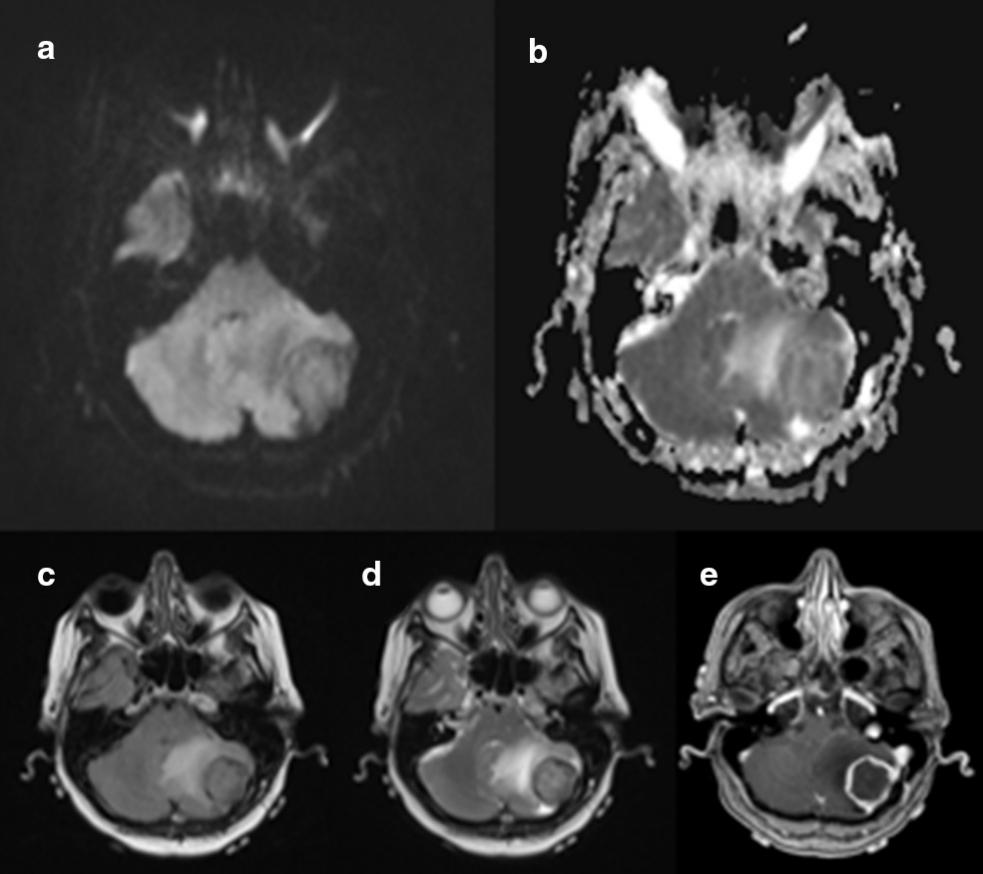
MRI scan of Case 1 demonstrating an extra-axial well-circumscribed mass within the left posterior fossa with a dural base against the left occipital bone. Diffusion-weighted image (DWI) (a) and associated apparent diffusion coefficientimage (b) demonstrate no restriction in diffusion. Fluid attenuation inversion recovery (c) and a *T*_2_ weighted image (d) shows associated increased signal consistent with peritumoral oedema and subsequent mass effect and effacement of the fourth ventricle. Contrast enhanced *T*_1_ image (e) shows a marginally enhancing lesion with dural tail.
